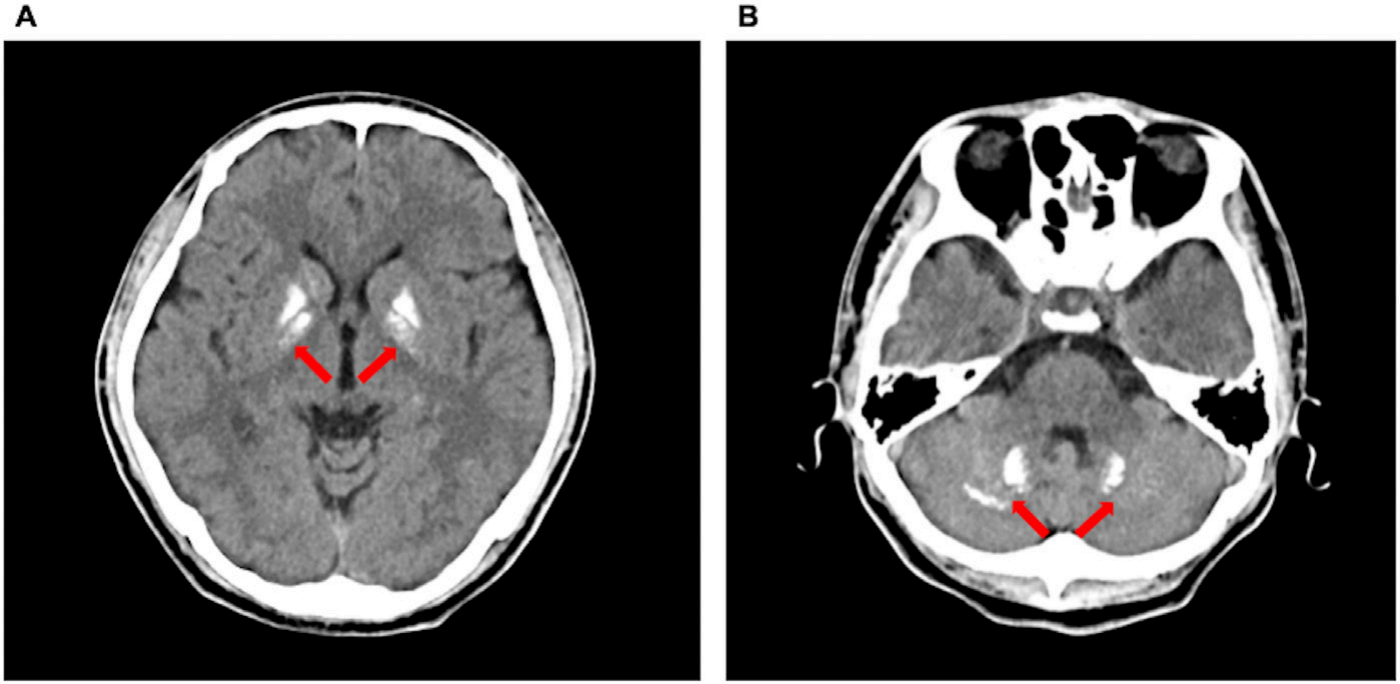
Non-contrast computed tomography showed intracranial calcifications (arrows) in Case 2, including bilateral basal ganglia (A) and bilateral dentate nuclei of the cerebellum (B).
